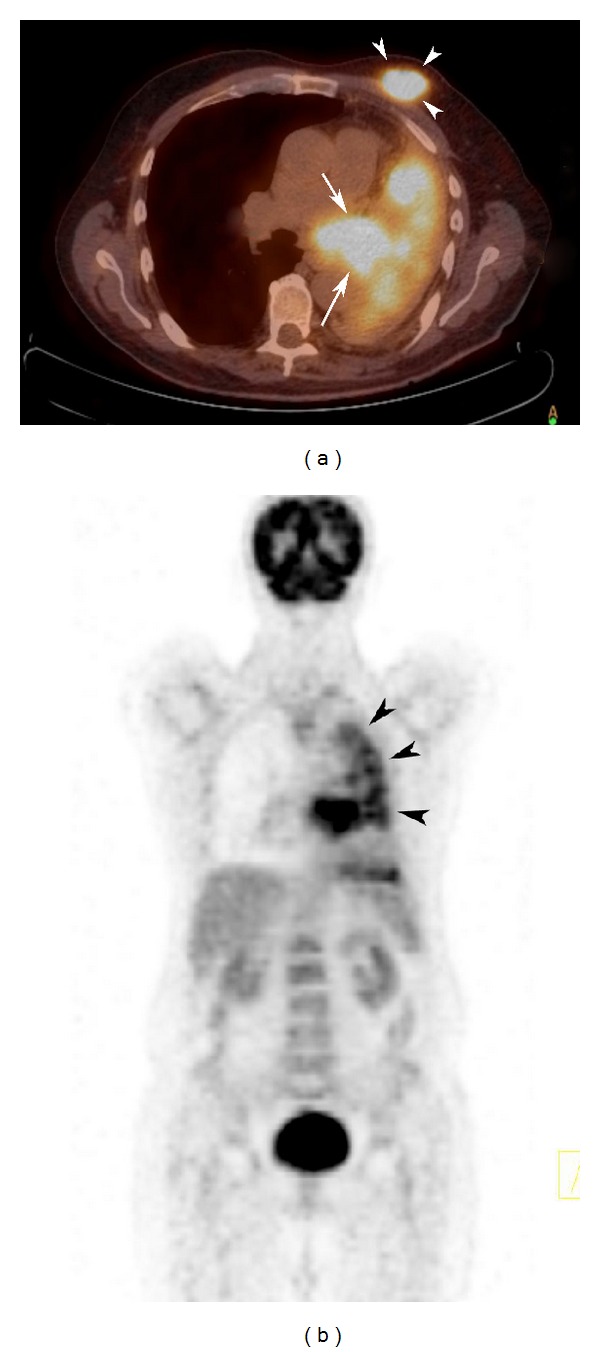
PET/CT demonstrates a primary lung cancer (arrows) at left hilar region and hypermetabolic masses with a high FDG uptake in left breast (arrow heads), consistent with metastasis (a). Whole body PET/CT was not positive for an FDG uptake suggesting metastasis in an organ other than the left breast. Nodular multiple high FDG uptake at the lateral part of the left lung was considered a false positive pleural uptake (arrow heads) (b).
